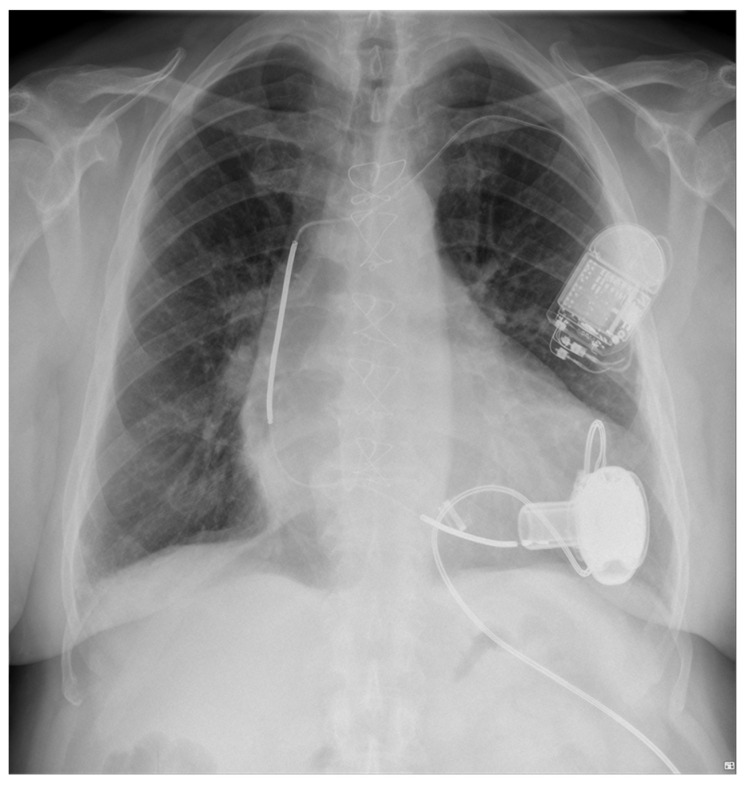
Chest X-ray. Posterior–anterior view. Patient with a transvenous single-chamber implantable cardioverter-defibrillator with a dual-coil lead and a left ventricular assist device. Very close proximity of the LVAD inlet cannula and the sensing bipole located on distal lead tip can be noted (structures overlapping).
